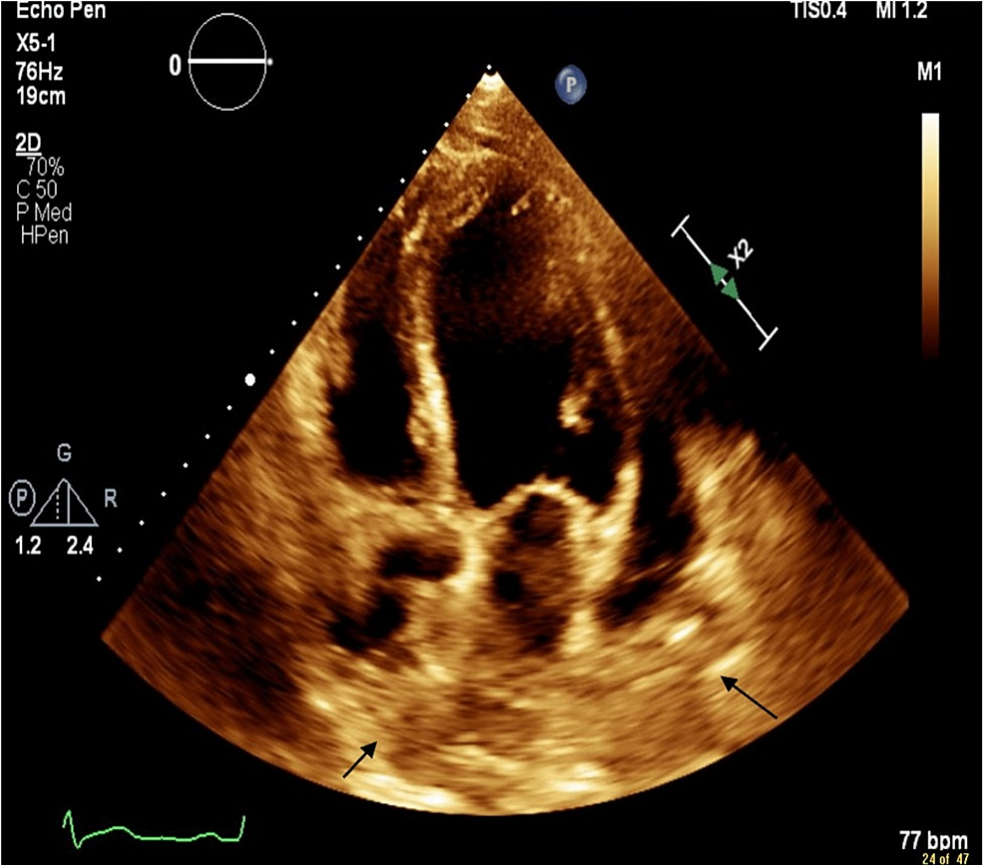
Echocardiogram apical four-chamber view (black arrows are pointing to the pericardial effusion)
